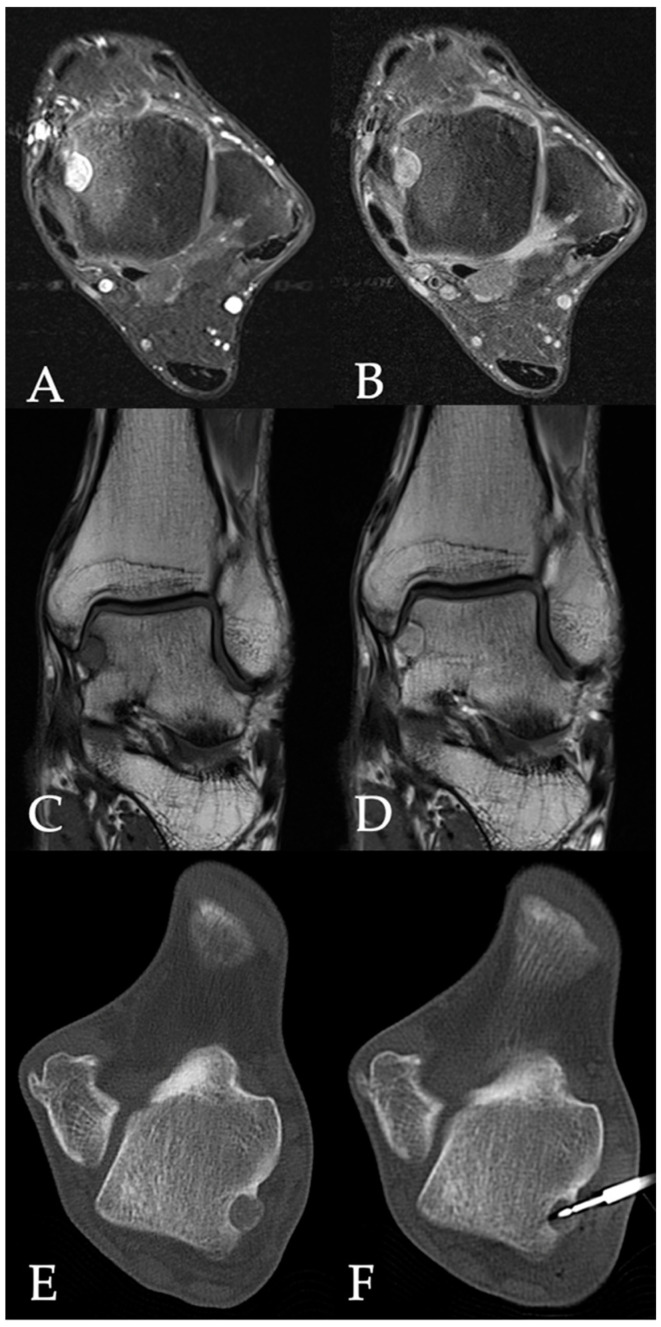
30-year-old male patient with an osteoid osteoma in the talus (A) [Axial proton density-weighted fat-saturated image], (B) [Axial contrast-enhanced T1-weighted fat-saturated image], (C) [Coronal T1-weighted image], (D) [Coronal contrast-enhanced T1-weighted image]. Peri-interventional CT images of the lesion before and during placement of radiofrequency ablation needle (E,F).
